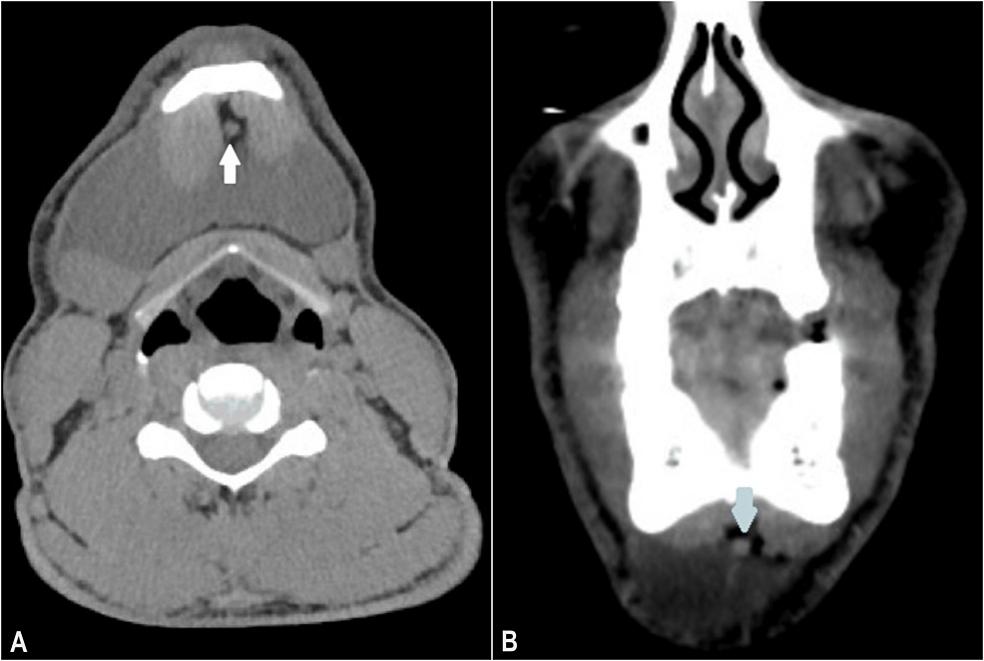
(A) Axial CT image showing the accessory lobe of the right sublingual gland (white arrow). (B) Coronal CT image showing accessory lobe of the right sublingual gland (blue arrow)
